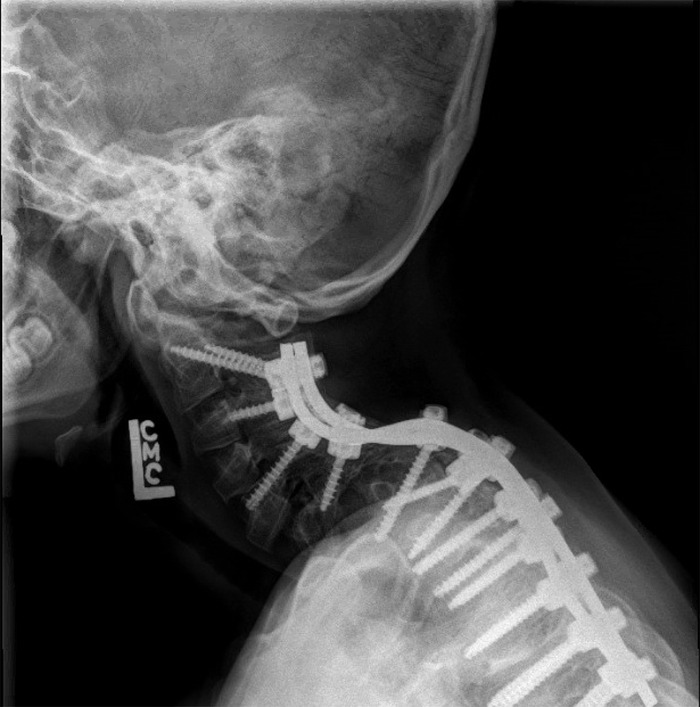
Lateral radiograph of cervical spine of the male sibling after undergoing posterior spinal fusion for scoliosis at age 5.
